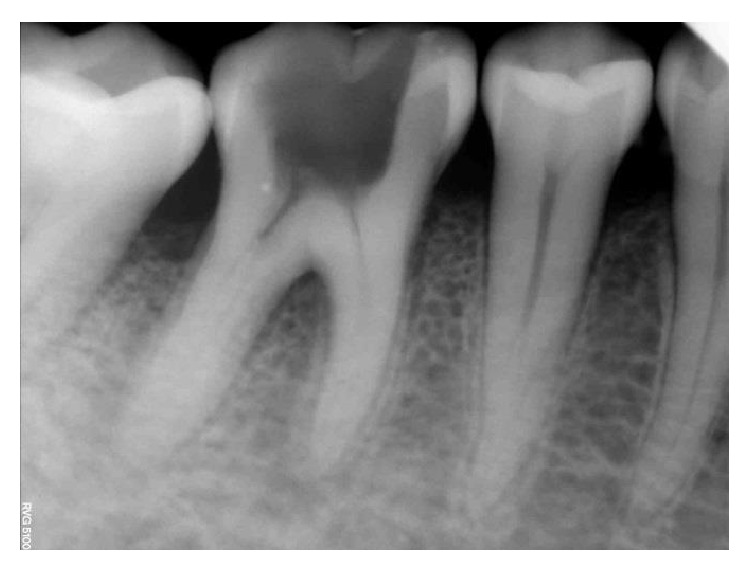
Radiograph showing the fracture of the cusp 36.
